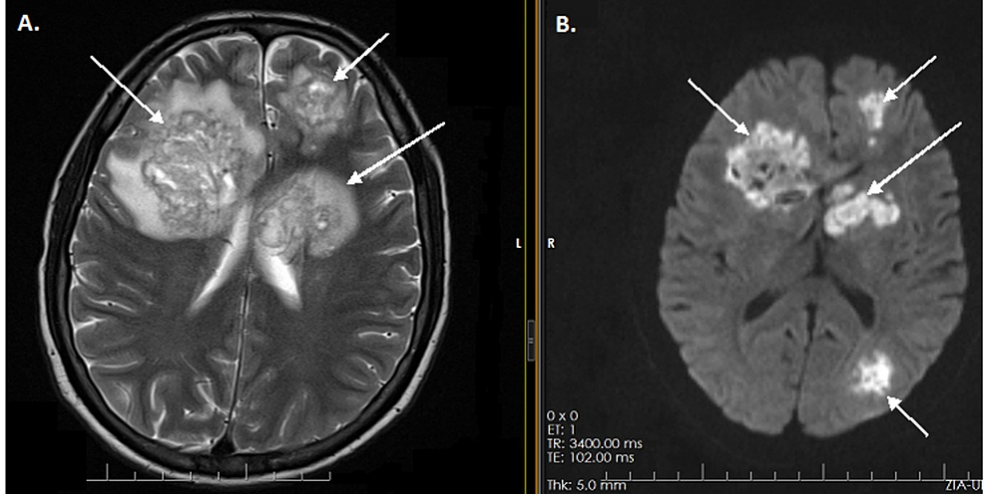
Diffusion-weighted magnetic resonance images of the brain with arrows pointing out multiple lesions with restricted diffusion in both cerebral hemispheres, including the basal ganglia, left thalamus, left cerebral peduncle, and right frontal lobe, surrounded with vasogenic edema (A, B)
